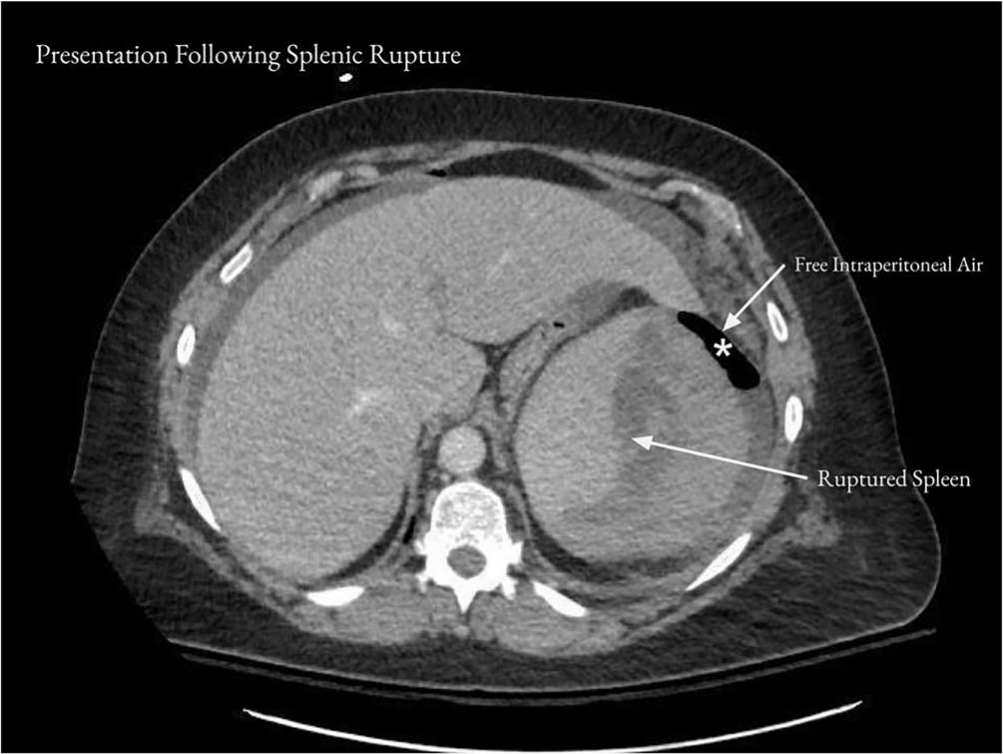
After the patient represented with acute worsening of abdominal pain, CT of the abdomen and pelvis showed a 7.2 cm subcapsular hematoma of the spleen (arrows) and moderate free intraperitoneal air (asterisk).
